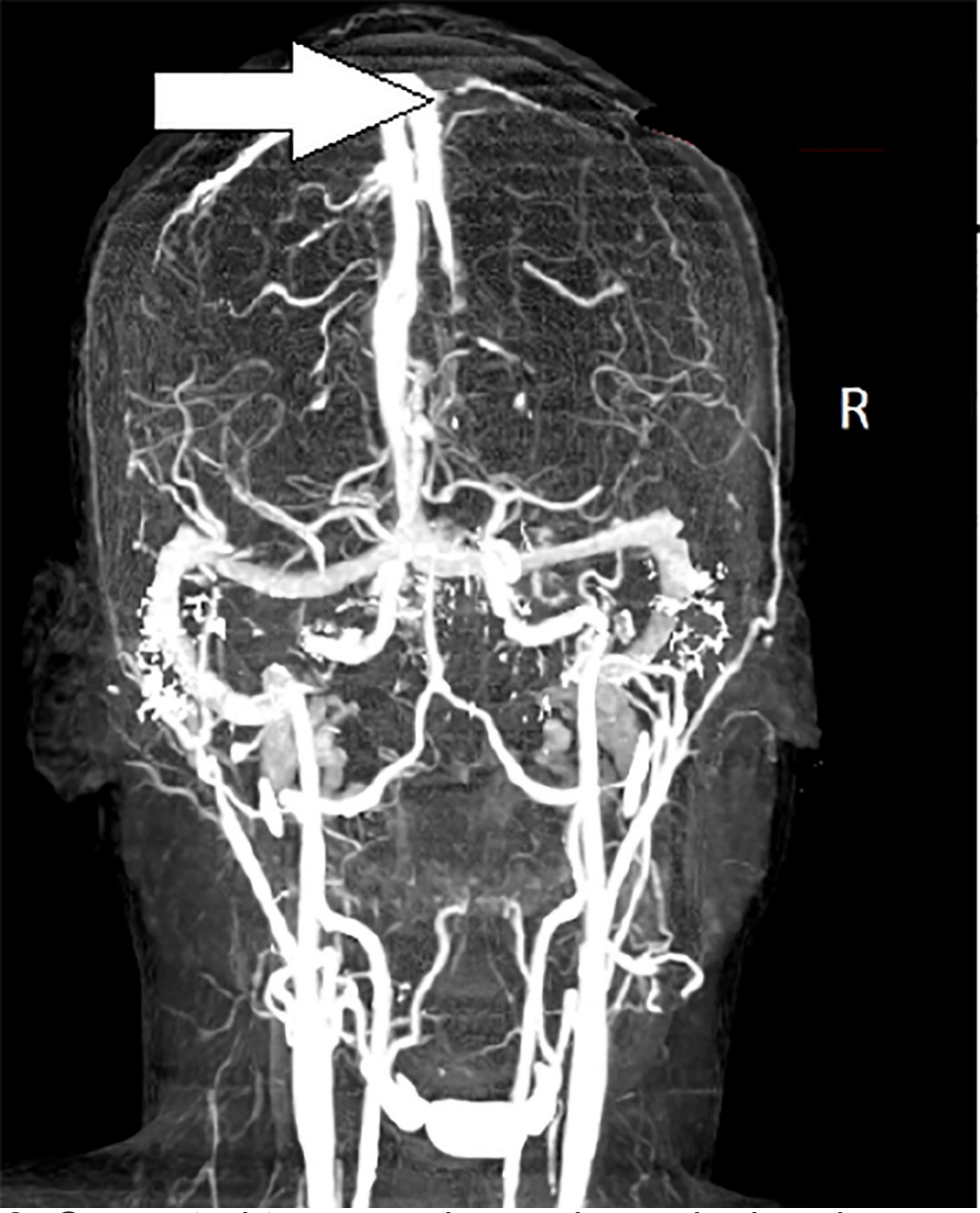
Computed tomography angiography head, coronal view demonstrating right middle cerebral artery (R-MCA) filling defect (arrow).
